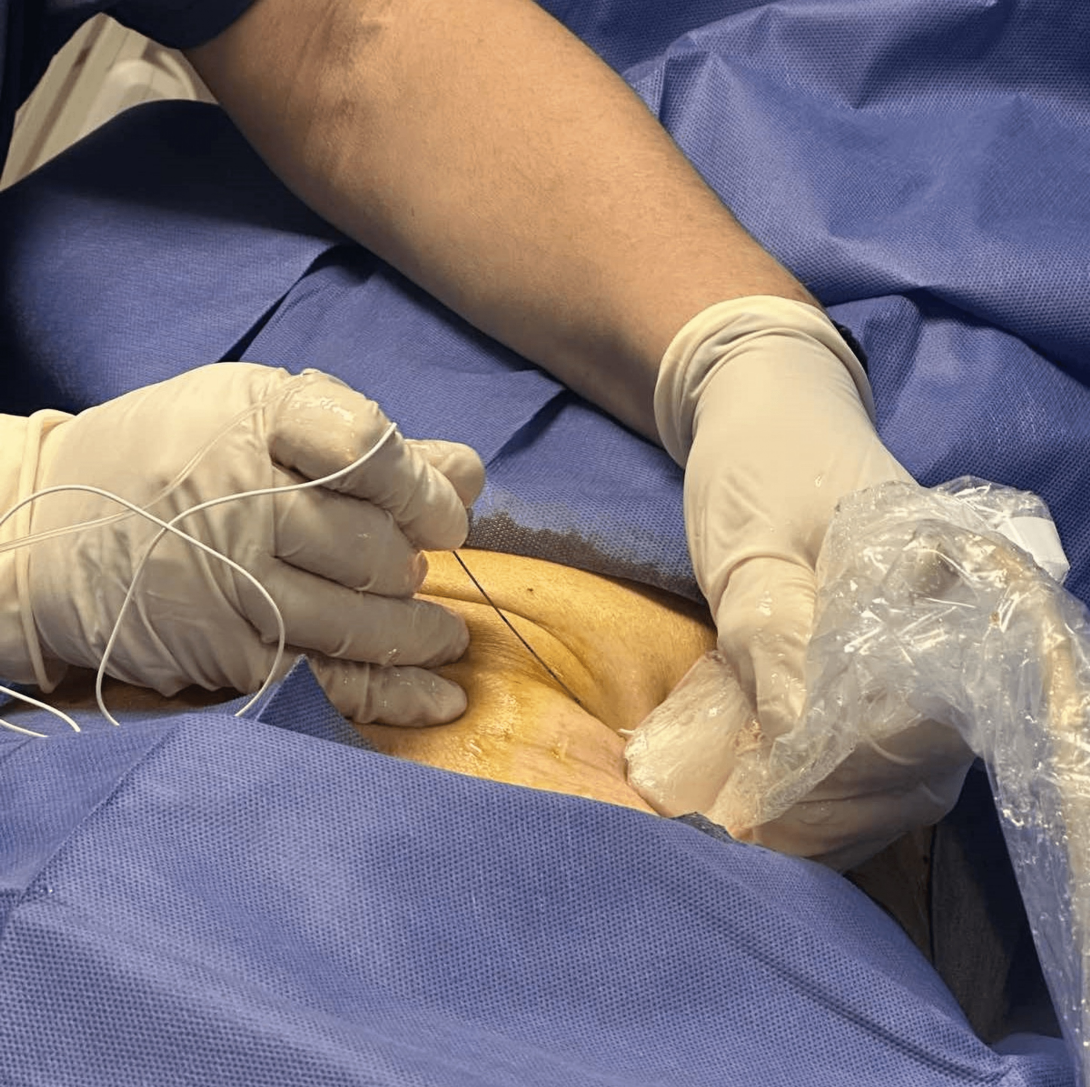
Ultrasound-guided ilioinguinal and iliohypogastric nerve block
